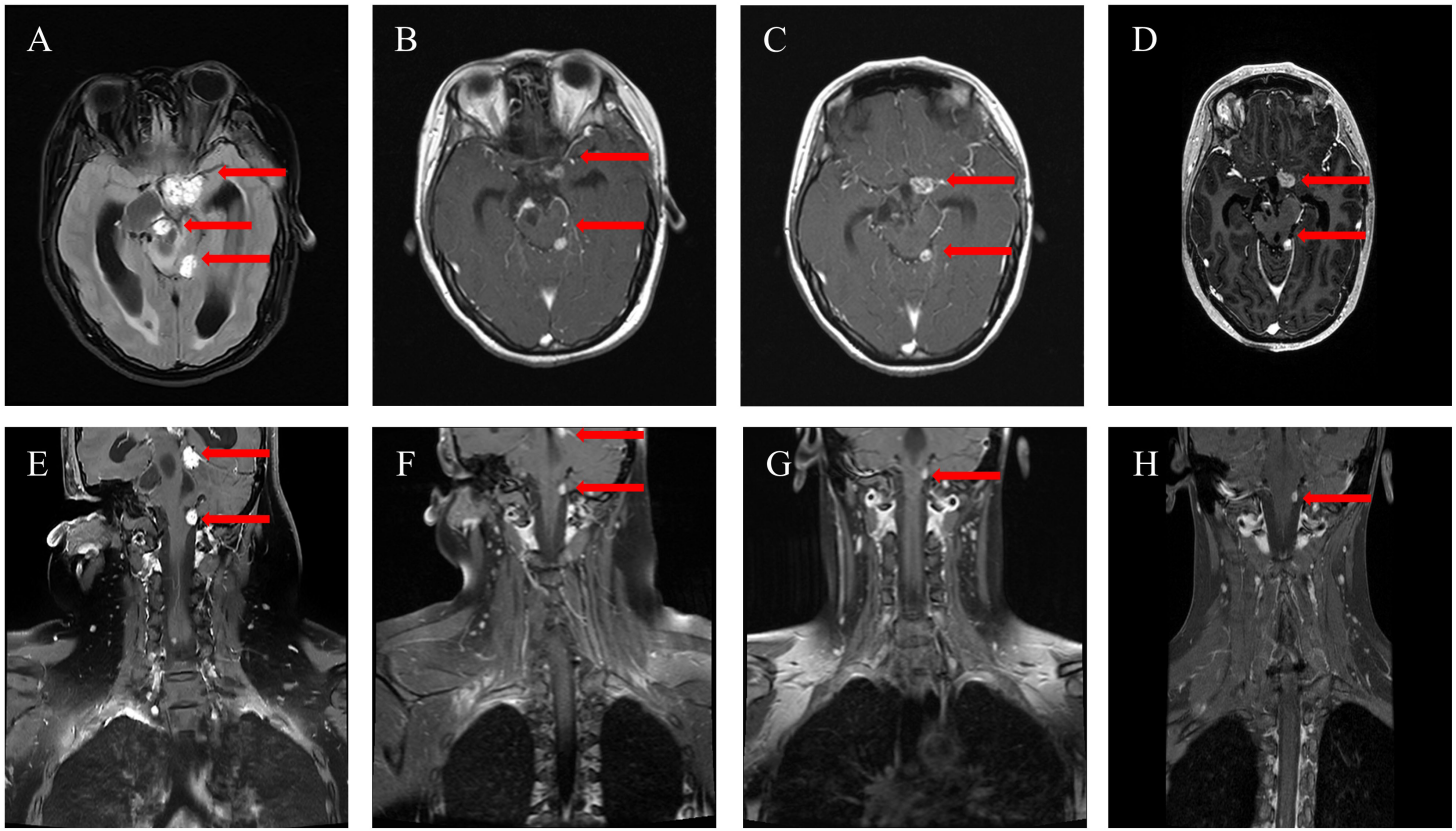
Changes of intracranial and spinal canal HBs of the MRI. (A, E) The lesions (red arrow) were observed in October 2023 before anlotinib treatment; (B, F) The MRI demonstrated tumor regression (red arrow) after three months of anlotinib in January 2024; (C, G) The MRI showed further tumor regression (red arrow) after seven months of anlotinib in May 2024; (D, H) The MRI showed continued stability (red arrow) after ten months of anlotinib on August 2024.
